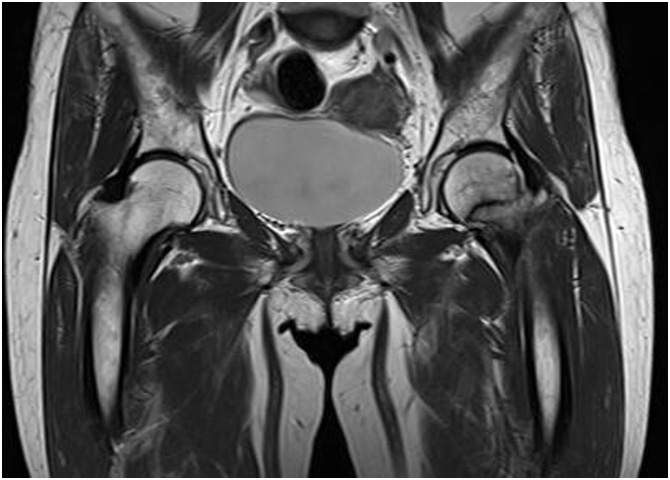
The MRI scan showed the incomplete linear fracture of the Lt. femoral neck.
